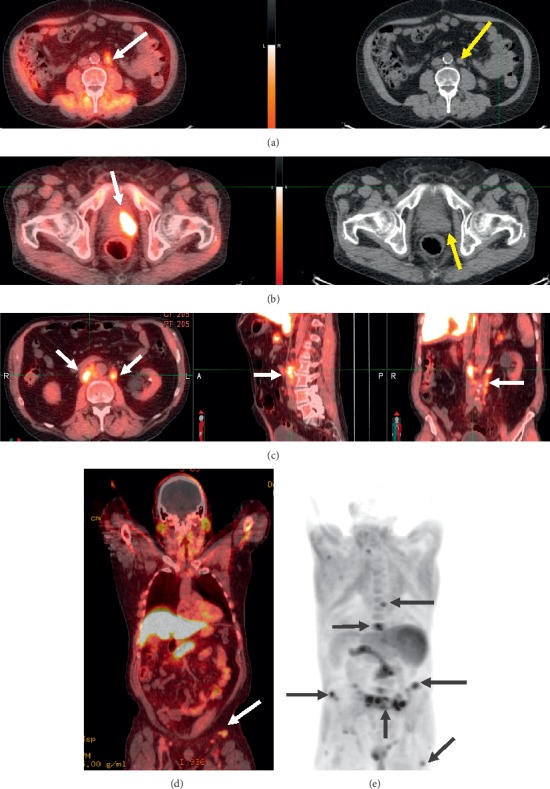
Elements recommended for determining likelihood of prostate cancer in given lesions (identified by arrows). (a) Comparison of fluciclovine uptake in nonosseous lesions to background; this is a para-aortic lymph node on fused axial image (left) and CT (right). (b) Lesion located at typical site of prostate cancer recurrence in the prostate gland in a patient given radiotherapy as initial definitive treatment (left image is axial fused and right image is corresponding CT). (c) Lesion located at typical site in lymph nodes (left image is axial fused, middle image is sagittal, and right image is coronal view). (d) Lesions located at atypical site for prostate cancer (lymph nodes). (e) Bone lesions as seen on an MIP image.
